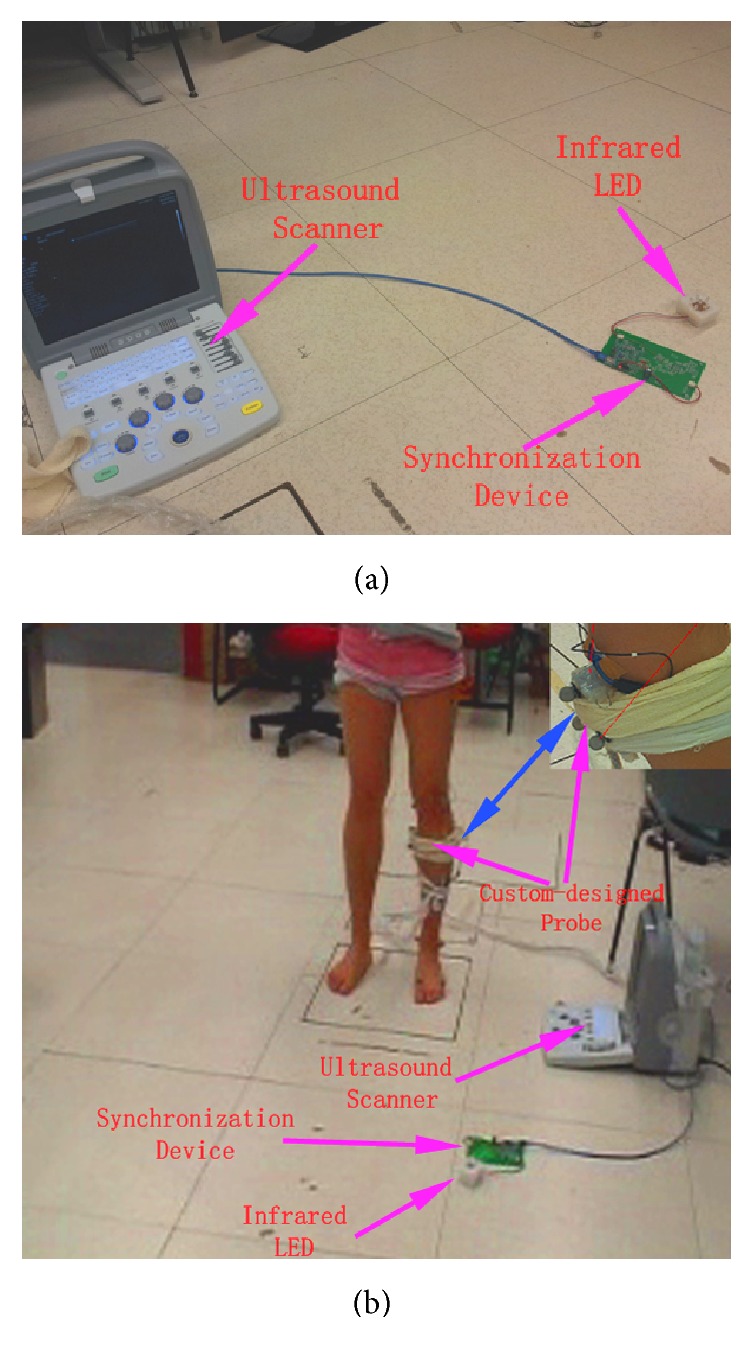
The ultrasound scanner with custom-designed probe and synchronization device: (a) the custom-designed synchronization device; (b) the custom-designed probe was attached on the muscle belly of GM.
